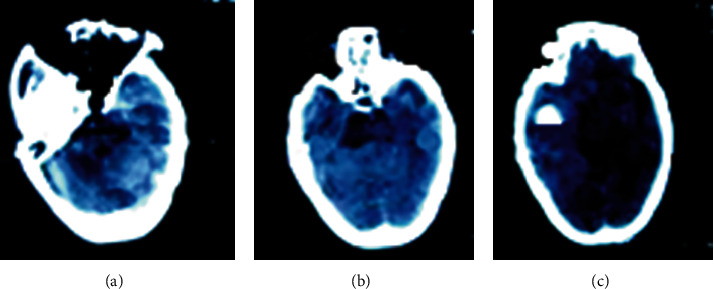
The images of routine CT examination after anesthesia of several patients. (a) showed the CT image of a male patient (aged 49 years old). (b) gave the CT image of a male patient (aged 65 years old). (c) revealed the CT image of a female patient (aged 53 years old).
